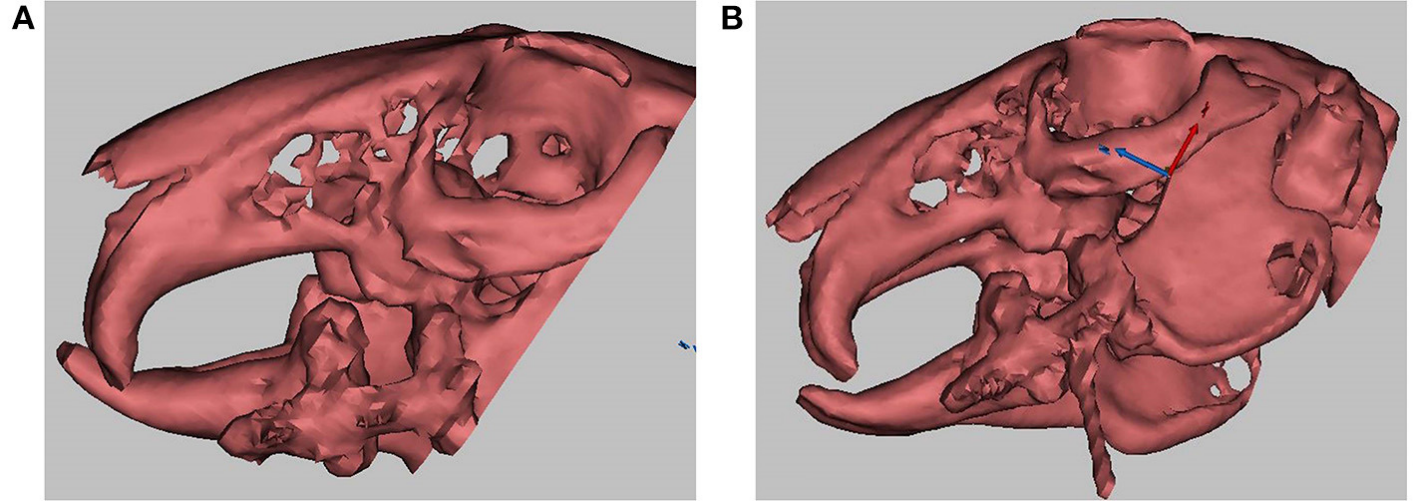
(A) demonstrates that the distraction length achieved the experimental expectation (1 cm), and the arrow indicates the distraction gap; (B) shows a real-time computed tomography (CT)-guided distraction injection, and the arrow indicates the syringe needle.
